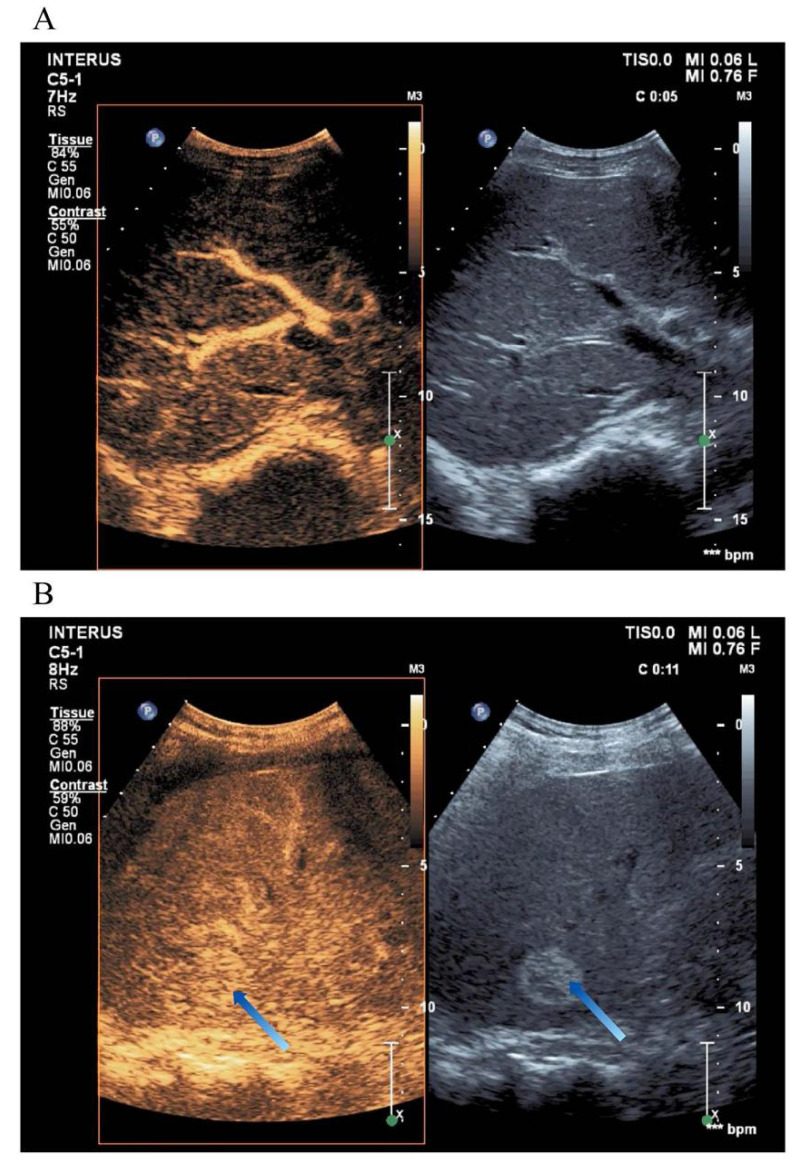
Liver Contrast-Enhanced Ultrasound Images. (A) CEUS provides a more detailed visualization of the liver's blood flow trajectory compared to conventional ultrasound imaging. (B) The arrow indicates a distinctly delineated, highly echogenic elliptical area, representing a liver hemangioma.
